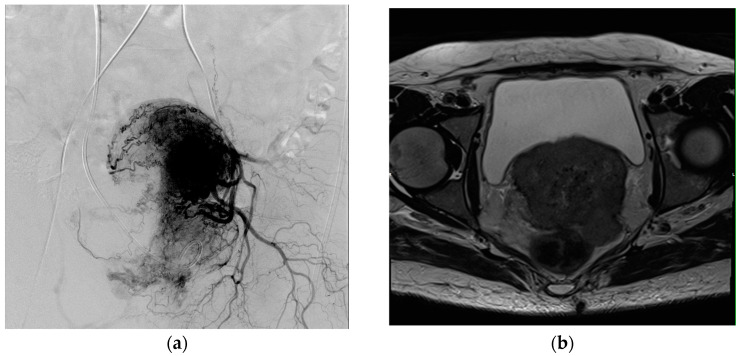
(a) Angiographic image of a transarterial chemoembolization (TACE) procedure in a patient with cervical carcinoma T4N2M1. The catheter was placed in the left internal iliac artery. (b) Transverse T2-weighted MRI image of the pelvis, in which the treated tumor is detectable.
